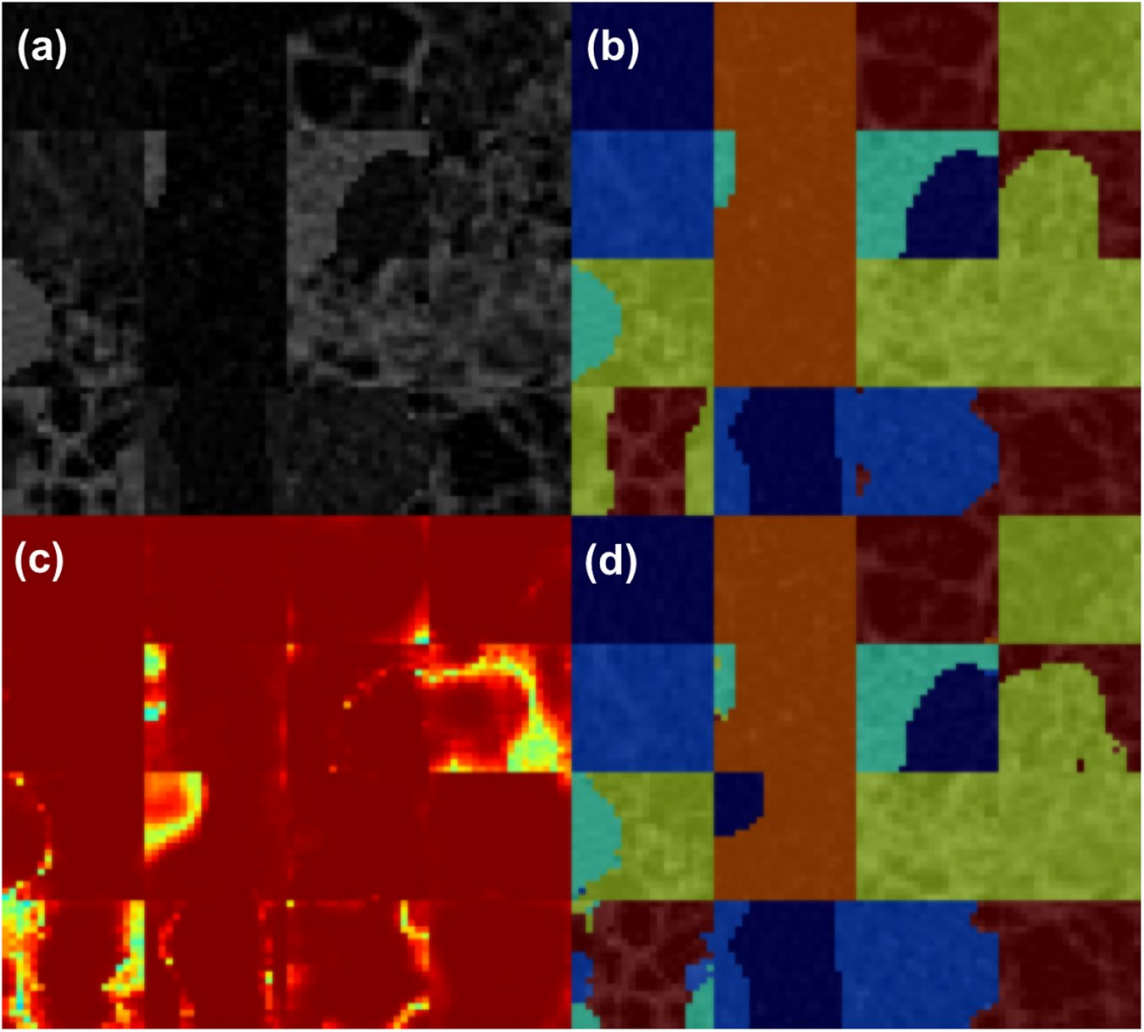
Examples of 10 ROIs (size of 20 × 20 pixel) of each class of DILD image patterns in HRCT. Examples of the CNN training process: (a) a batch of 16 ROIs generated using Perlin noise, (b) corresponding masks representing the DILD classes for the batch; normal (dark blue), GGO (light blue), consolidation (cyan), RO (yellow), emphysema (orange), and honeycombing (brown), (c) softmax probability maps for the batch; high probability (red), low probability (green), and (d) predicted labels; color scheme is the same as (b). We note that the figure is best viewed in color.
